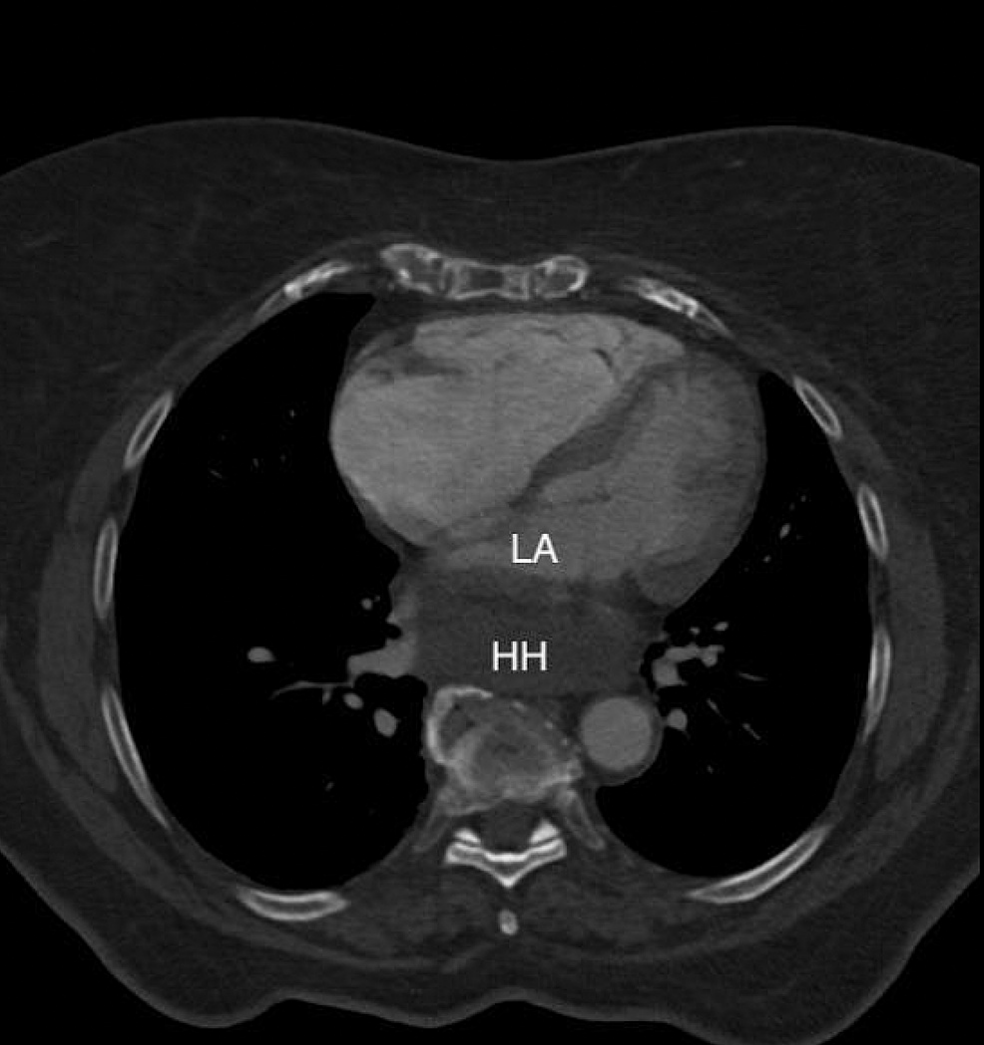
Alternate axial view of CT chest with compression of left atrium by a giant hiatal hernia. LA: left atrium; HH: hiatal hernia
